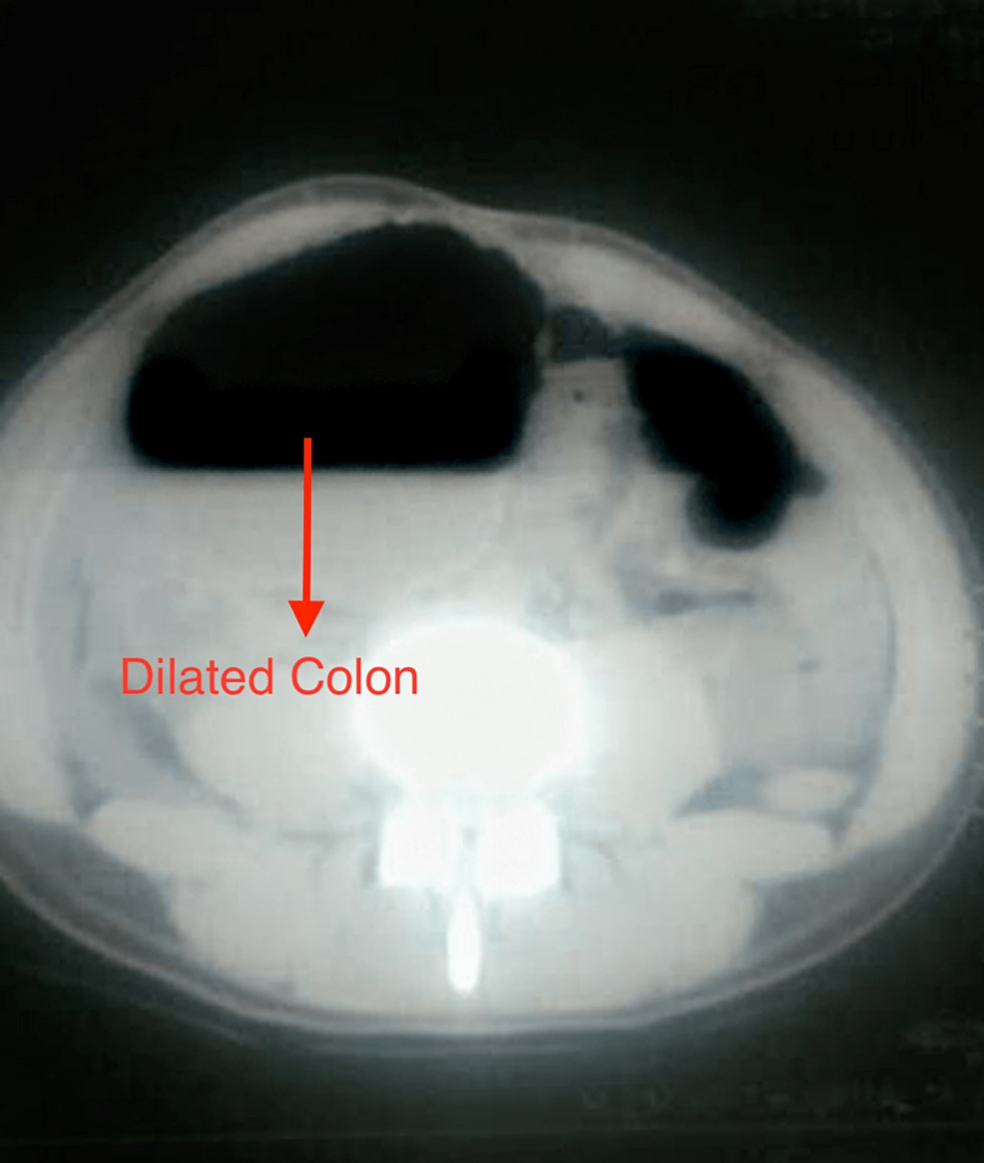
CT finding showing dilated large bowel on the right side
